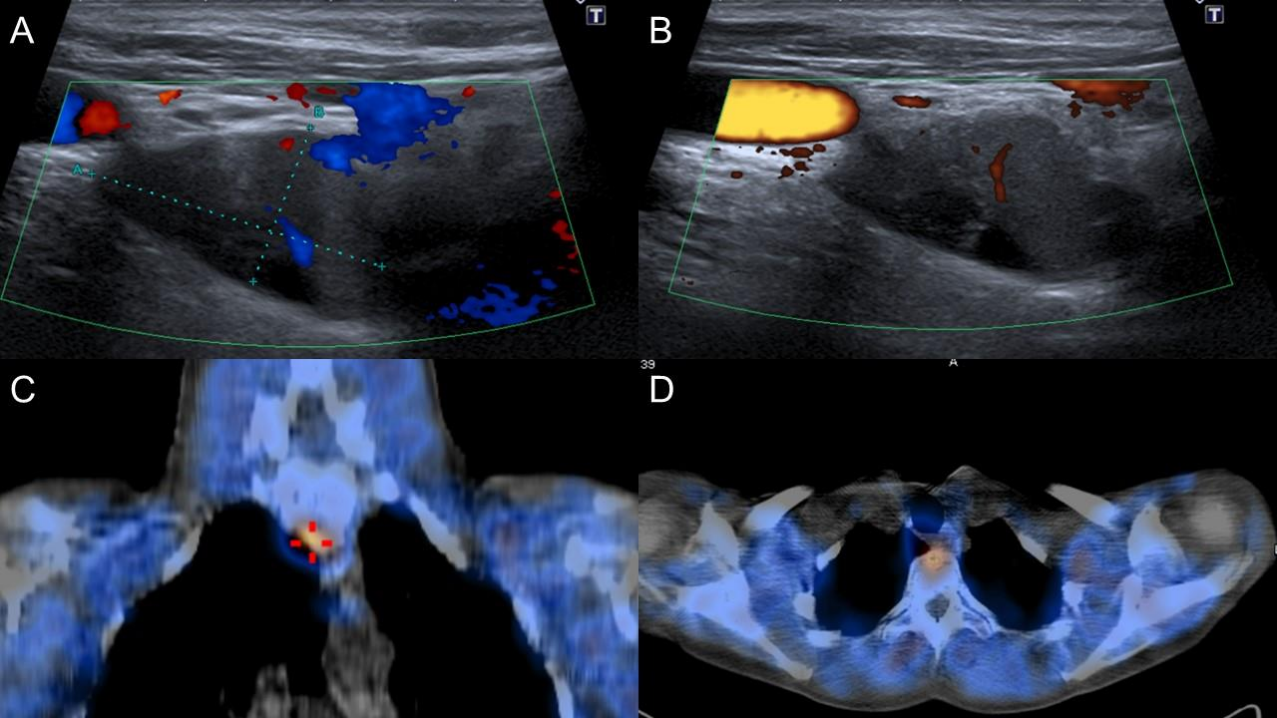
Preoperative parathyroid localization studies. A and B. Parathyroid ultrasound (sagittal orientation). Adjacent to the lower pole of the right lobe, a mixed, solid formation of approximately 24 x 13 mm is observed, which shows central flow on color Doppler. C and D. 99mTc-sestamibi scintigraphy with SPECT [coronal (C) and axial orientation (D)]. Focus of intense tracer-uptake, located below the right thyroid lobe, behind the trachea, and in front of the spine.
